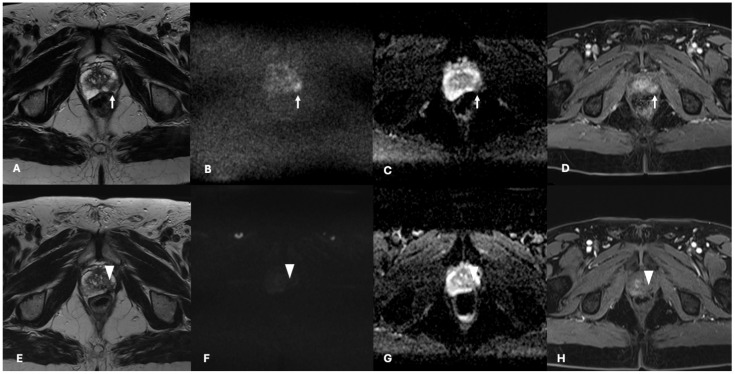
mpMRI assessment of lesions within the ablation zone after TPLA: a case of complete response. Top row ((A) T2 axial imaging, (B) DWI b = 1400 s/mm^2^, (C) ADC map, (D) dynamic contrast imaging) shows a PI-RADS 4 lesion (11 × 4 mm—white arrow) in the lateral aspect of the left peripheral zone of the prostate. Biopsy confirmed to be a Gleason Grade 7 (3 + 4), ISUP 2, treated with TPLA focal therapy. Bottom row ((E) T2 axial imaging, (F) DWI b = 1400 s/mm^2^, (G) ADC map, (H) dynamic contrast imaging) at 12-month follow-up; no residual cancer (white arrowhead) was detected, thus the score for this area is TARGET 1.
